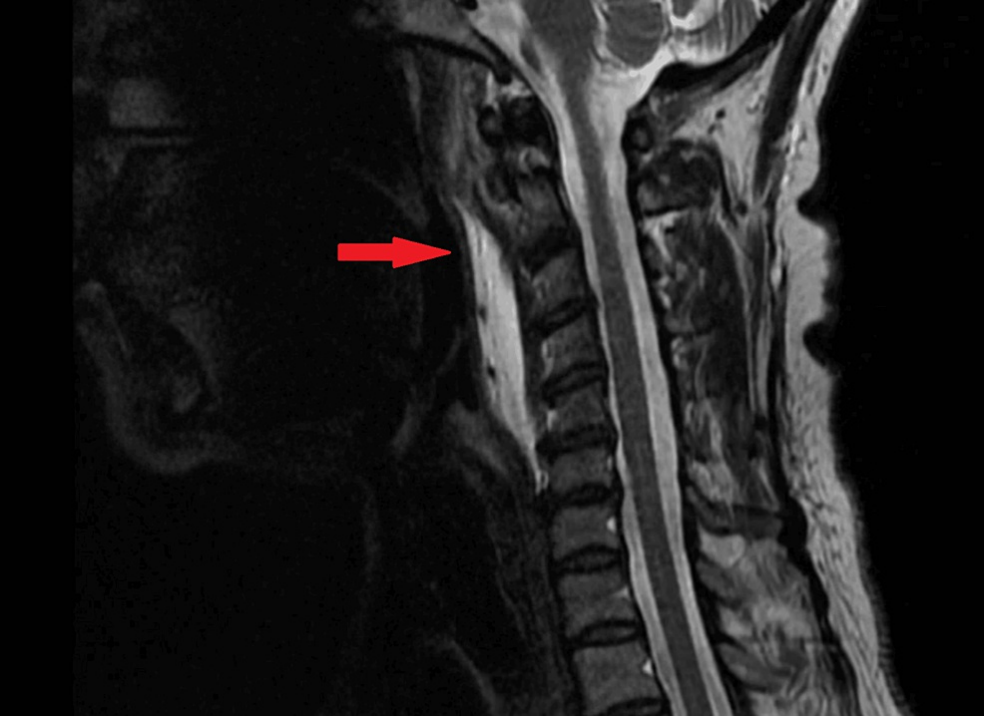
Magnetic resonance imaging (MRI), coronal T2-weighted image of the neck Retropharyngeal effusion (red arrow)
